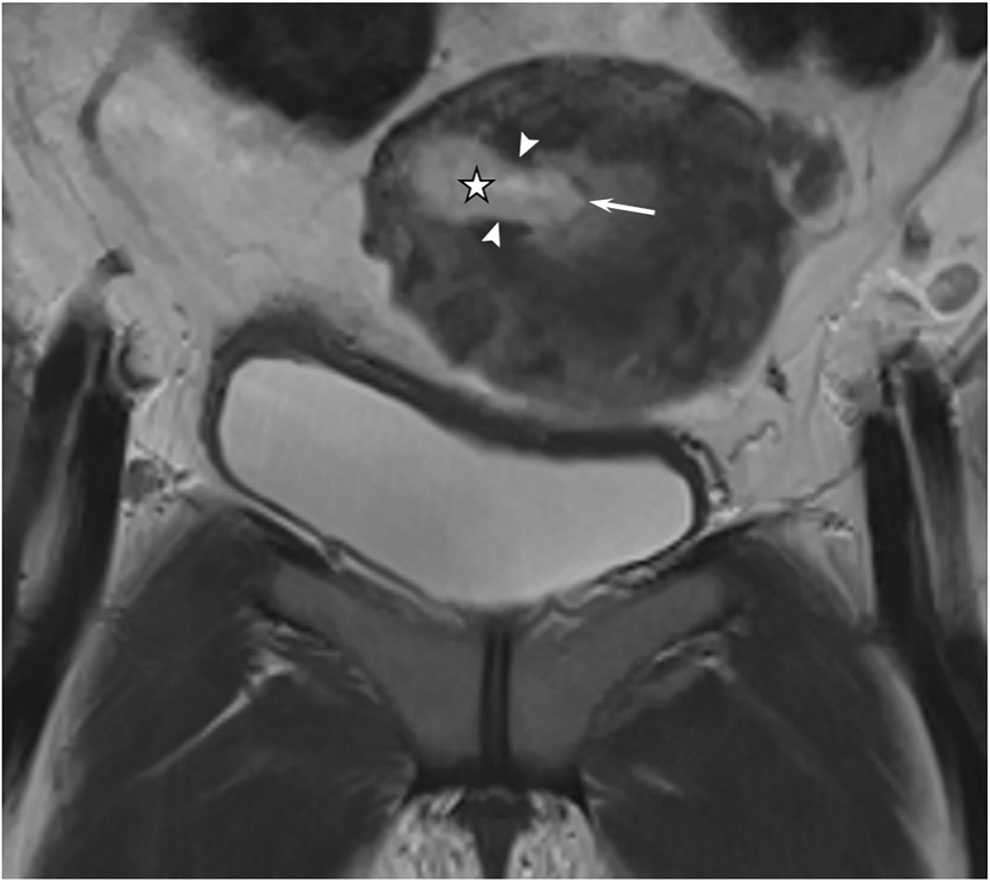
MRI in a 30-year-old patient with interstitial pregnancy. Coronal T2-weighted image showing the gestational sac (star), medial free edge (arrow), and interrupted lateral junctional zone (arrowheads)
